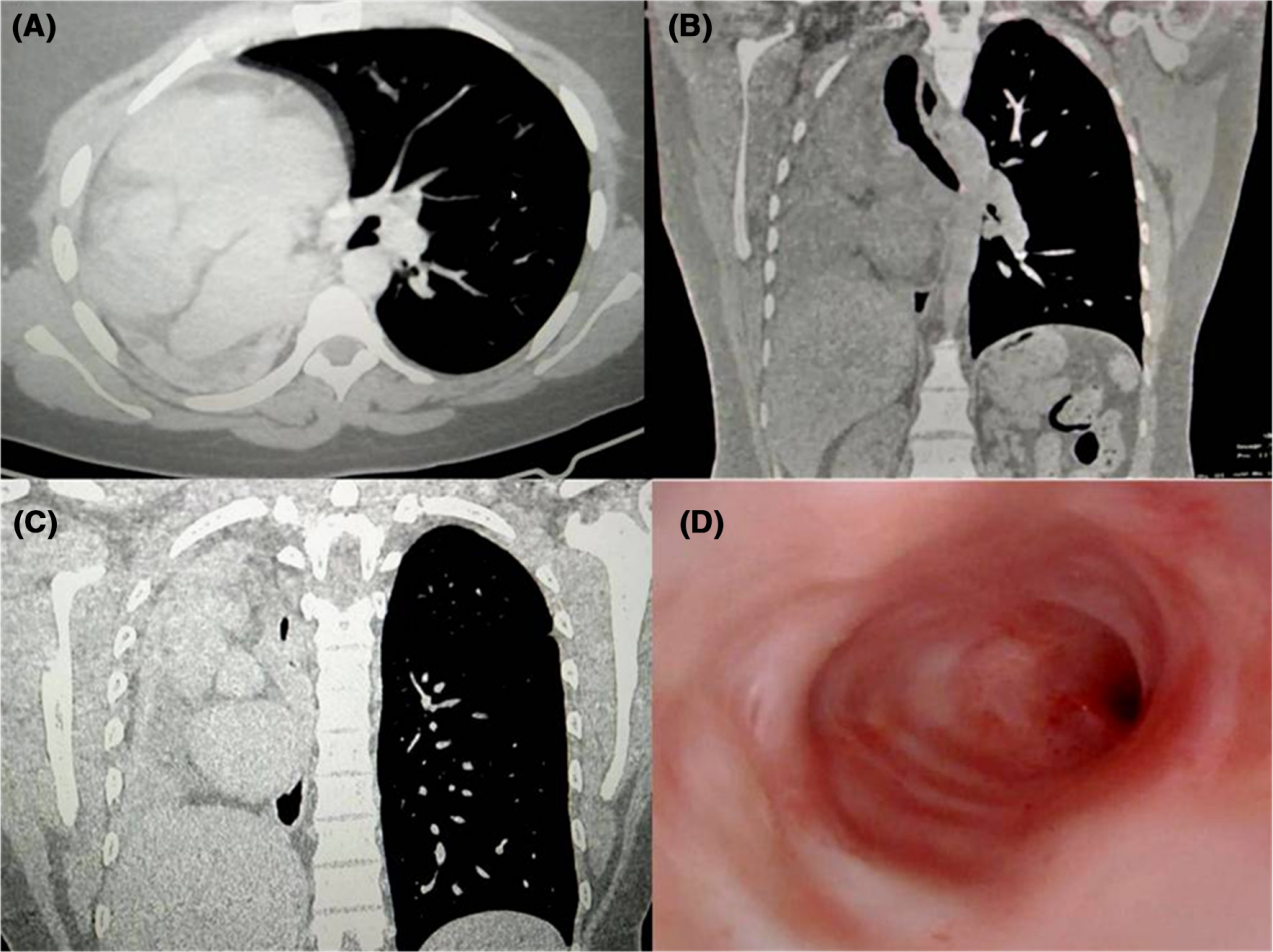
(A–C) Chest computed tomography, axial (A), and coronal (B and C) planes: Absence of right lung parenchyma, bronchial and vascular structures, with right‐sided herniation and displacement of the mediastinal structures to the right. Bronchofibroscopy (D): Absence of the carina and right main bronchus, with preserved left bronchial tree structures.
